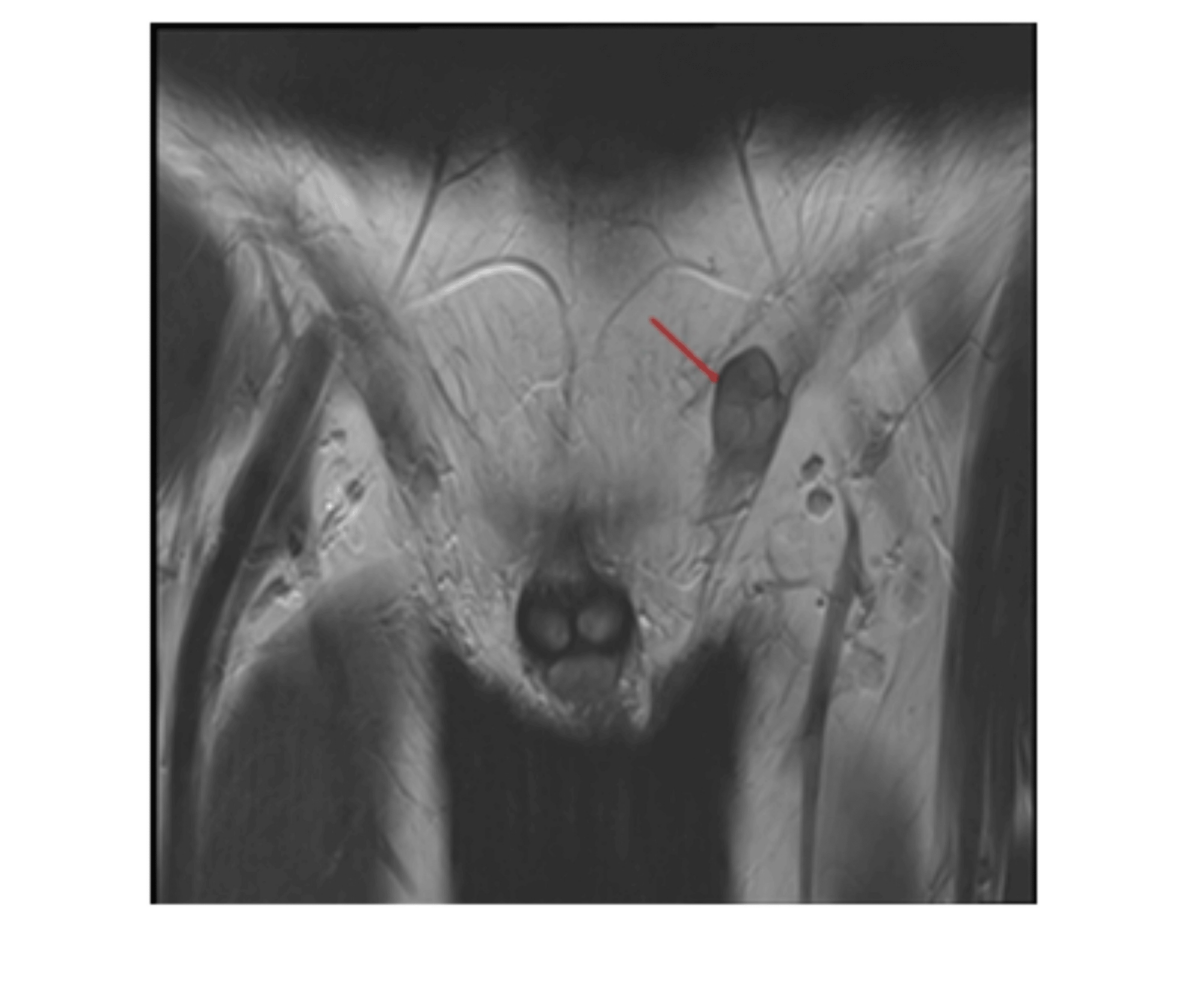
Coronal T2-weighted image MRI scan of the pelvis shows a small heterogeneous mass, with iso- and high T2 signal which raised the suspicion of hypoplastic testicle against Müllerian duct derivatives (further described below). This figure is the original work of the authors. Patient consent for the use of the image was obtained, as mentioned in the patient consent form.
